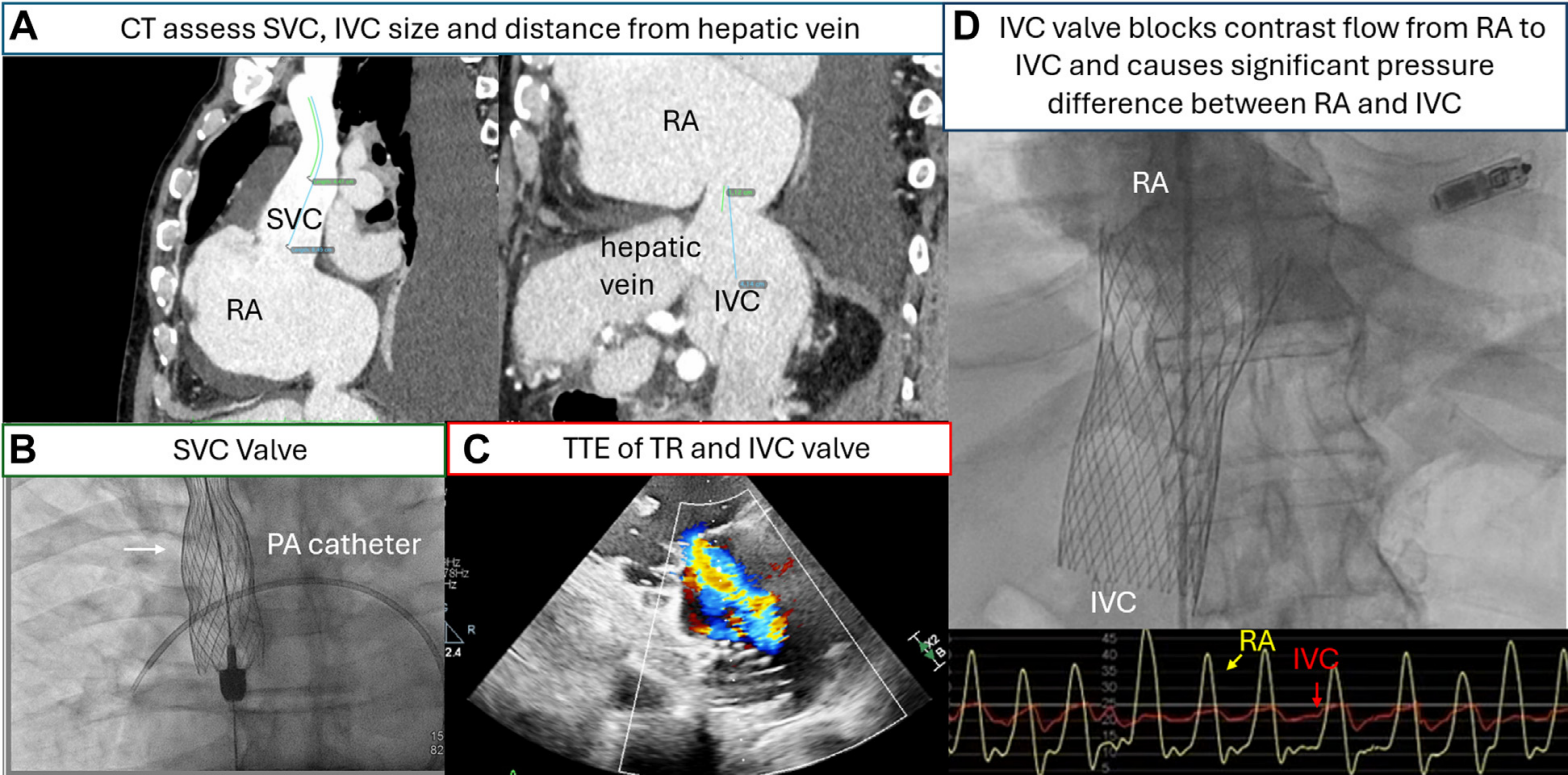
Multimodality Imaging to Guide TricValve Heterotopic Transcatheter Tricuspid Valve Replacement (A) Preoperative CT was performed to assess the sizes of SVC, IVC, and the distance of right atrium to the first hepatic vessel. (B) Implantation of the SVC valve with the belly (white arrow) of the valve positioned superior to the pulmonary artery additionally confirmed by a pulmonary artery (PA) catheter. (C) TTE showing the IVC valve in-situ with the presence of severe tricuspid regurgitation (TR). (D) The implanted IVC valve blocks contrast flow from right atrium (RA) to IVC and causes significant pressure gradient between RA and IVC. Abbreviaitons as in Figures 1 and 3.
